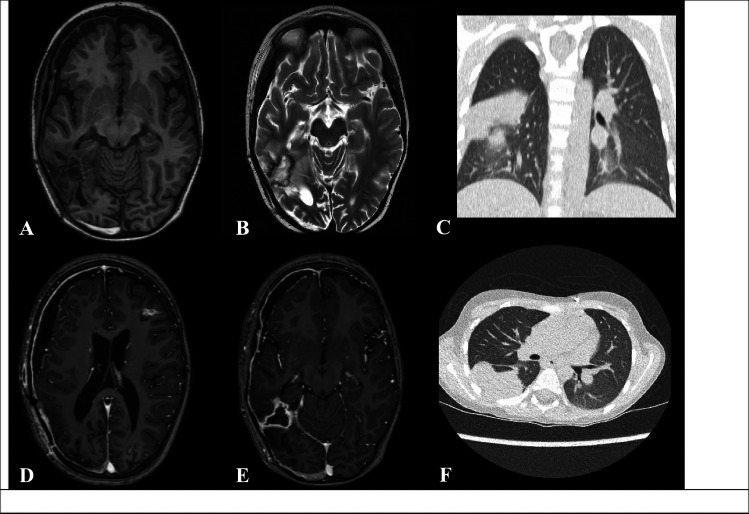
Magnetic resonance imaging of the head and computed tomography scan after two chemotherapy cycles. Axial images demonstrating very good partial response with expected postoperative changes. A T1-weighted image. B T2-weighted image showing oedema resolution post tumor resection. D Contrast-enhanced T1-weighted image. E Contrast-enhanced T1-weighted image demonstrating interval reduction in the size of a separate metastatic lesion under ongoing chemotherapy, indicating a favorable treatment response. C coronal and F axial CT-thorax images
